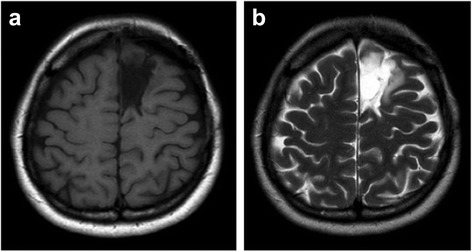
Axial sections of magnetic resonance imaging scan performed 11 months after awake craniotomy. T1-weighted image (a) and T2-weighted image (b) show no evidence of tumor recurrence
